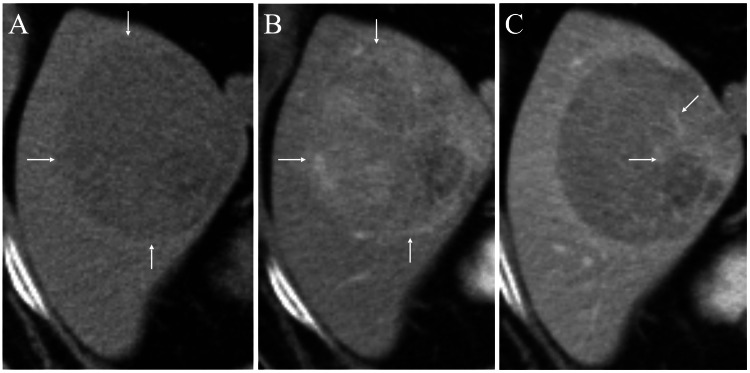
Computed tomography (CT) findings (A) Plain CT showed an oval mass (arrows), which had clear margins and a slightly lower Hounsfield Unit value than that of the surrounding liver parenchyma. (B) Enhanced CT showed a weak and heterogeneous early enhancement of the mass (arrows). (C) Enhanced CT showed a heterogeneous wash-out pattern of the mass and partially retained enhancement (arrows).
